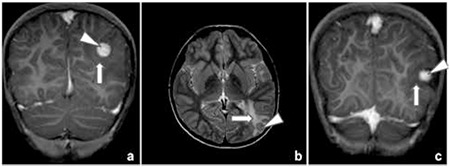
Coronal contrast-enhanced T1-weighted (a) and axial T2-weighted (b, c) magnetic resonance image shows two homogeneously enhancing lesions with surrounding vasogenic edema in the cortical-subcortical region of the left parietal and temporo-occipital lobes
